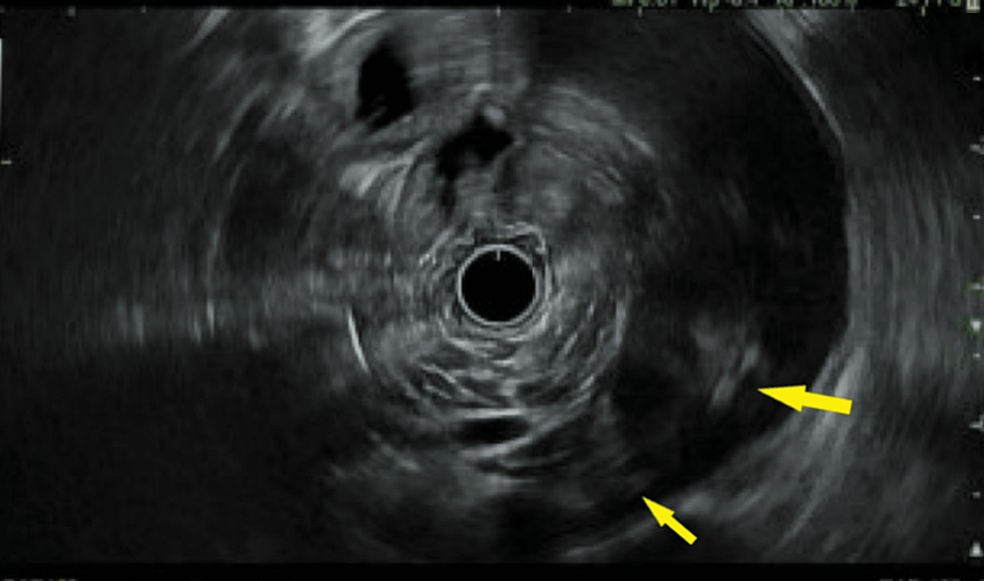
Initial EUS of the pancreas demonstrating moderate-to-severe chronic pancreatitis. Shown is the initial EUS demonstrating moderate-to-severe chronic pancreatitis. Arrows indicate chronic calcifications and heterogeneity of the pancreas. EUS: endoscopic ultrasound.
